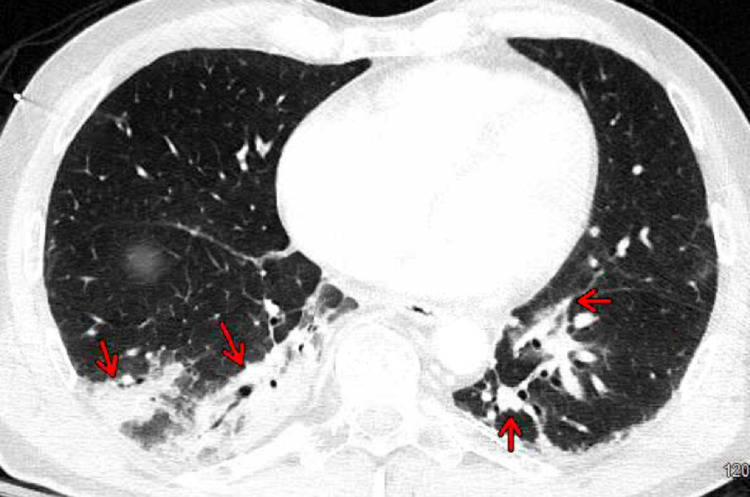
Computed tomography of the chest with contrast axial view revealed bibasilar right greater than left consolidations and ground-glass opacities (red arrows) with subsegmental atelectasis suggestive of pneumonia.
